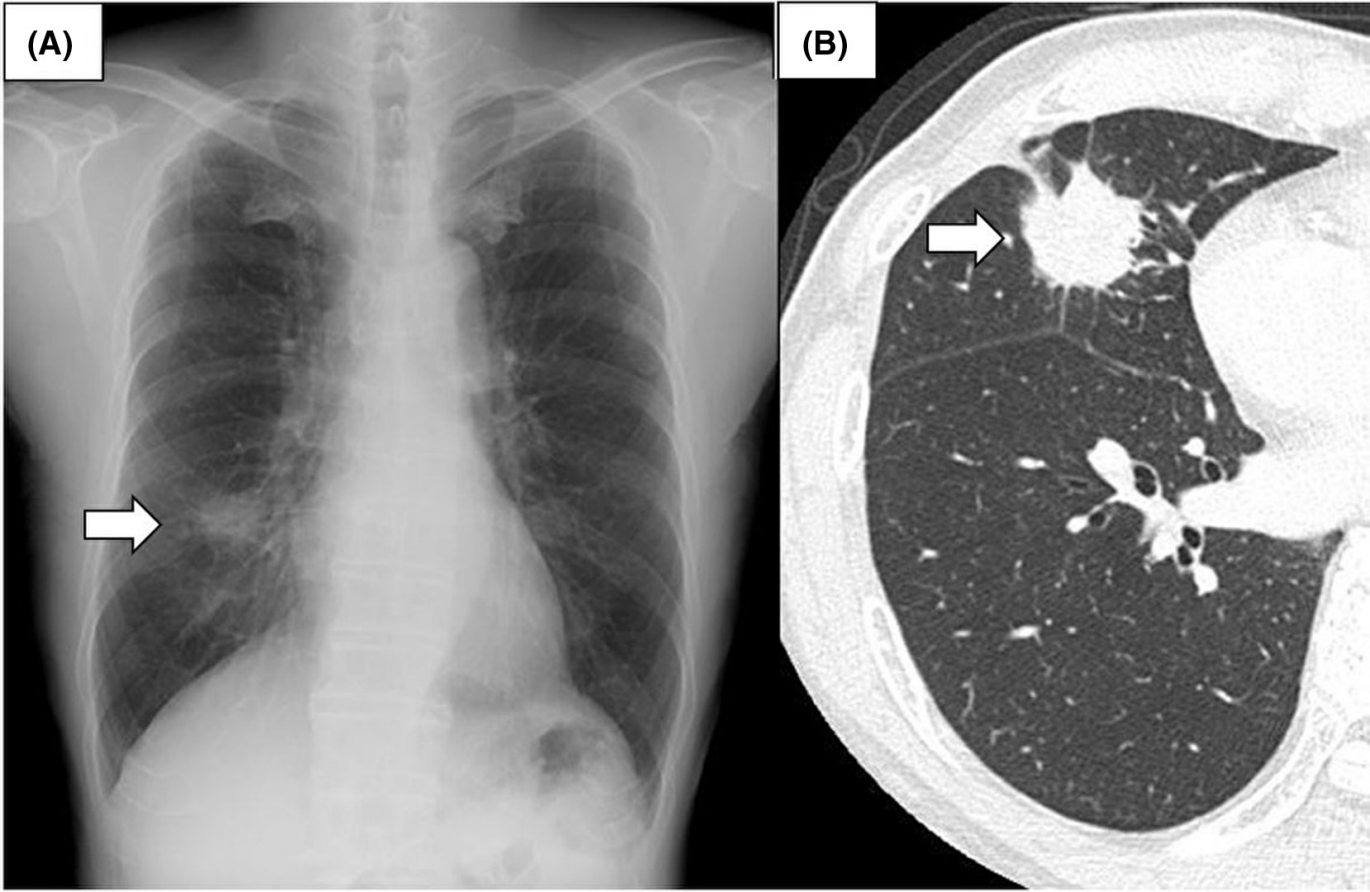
Imaging performed at the time of referral to our hospital showing a pulmonary tumor located in the lower lobe of the right lung. (A) Chest radiography showing a pulmonary nodule in the right lower lung area (white arrow). (B) Transverse view of chest computed tomography showing a pulmonary nodule in the middle lobe of the right lung (white arrow)
